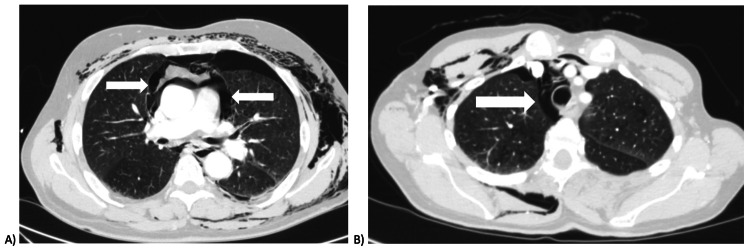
CT images of patients within our dataset Pneumomediastinum is highlighted by the white arrows. Note the subcutaneous emphysema and pneumothoraces in both patients (A, B).
